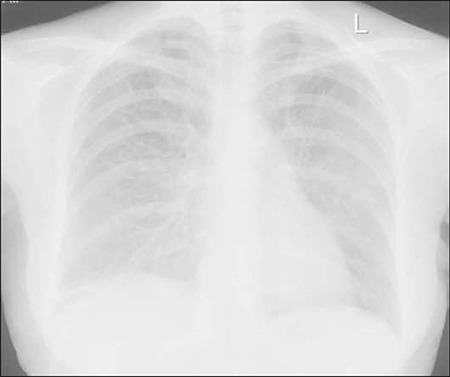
Chest X-ray showed complete resolution of diffuse interstitial changes after prednisolone therapy.
